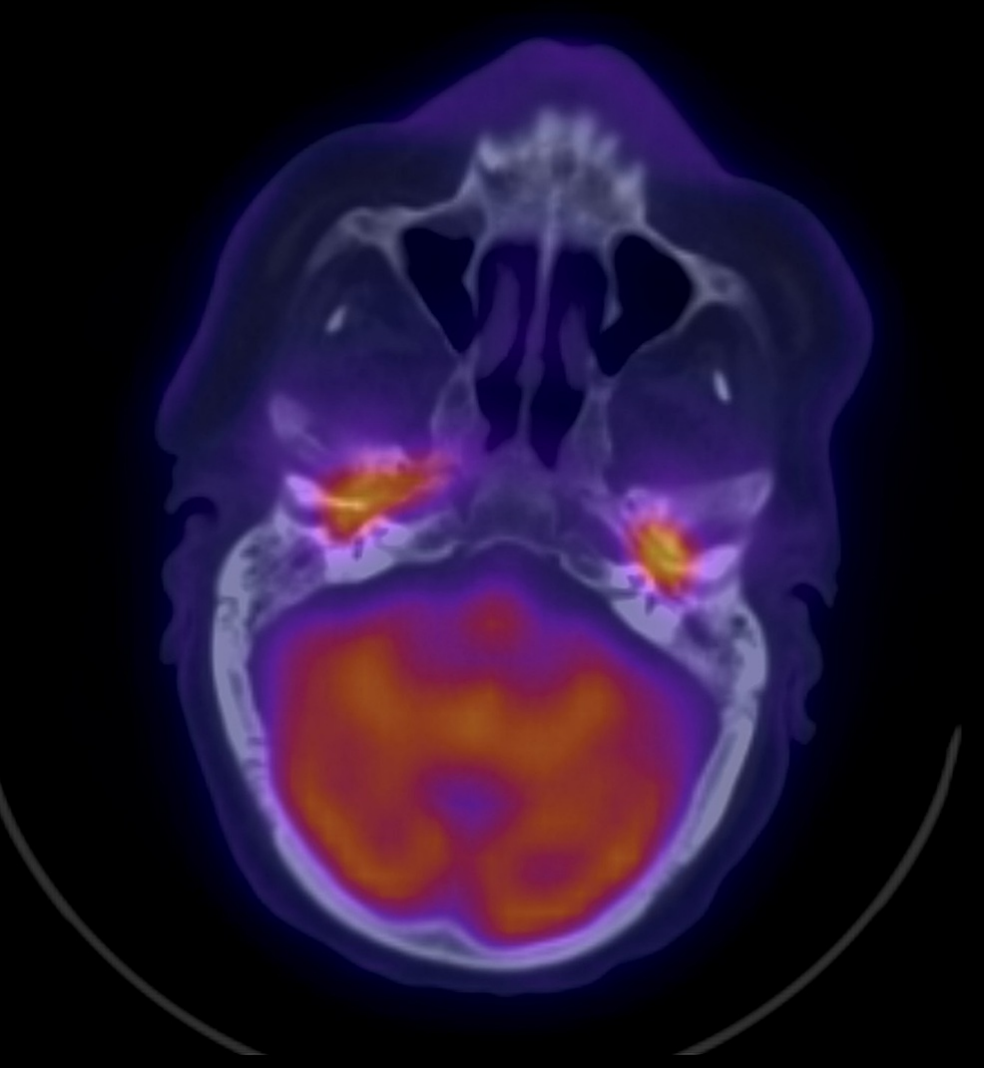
Fused transaxial F-18 FDG-PET/CT showing bilateral hypermetabolic involvement of both middle ears without direct extension from one side to the other through the skull-base structures FDG-PET: fluorodeoxyglucose-positron emission tomography
